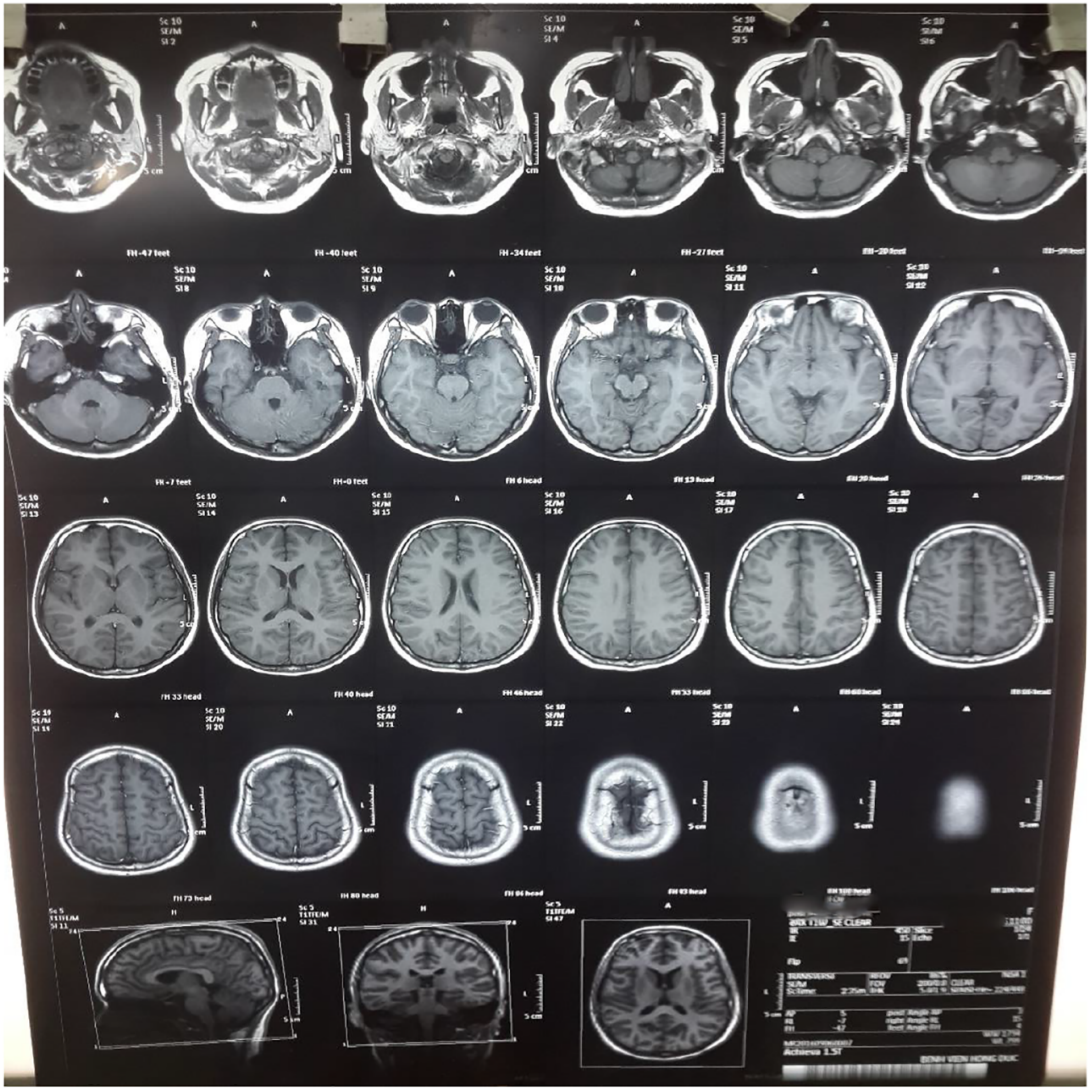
Magnetic resonance imaging (MRI). Axial non-contrast (fluid-attenuated inversion recovery (FLAIR) MRI.
